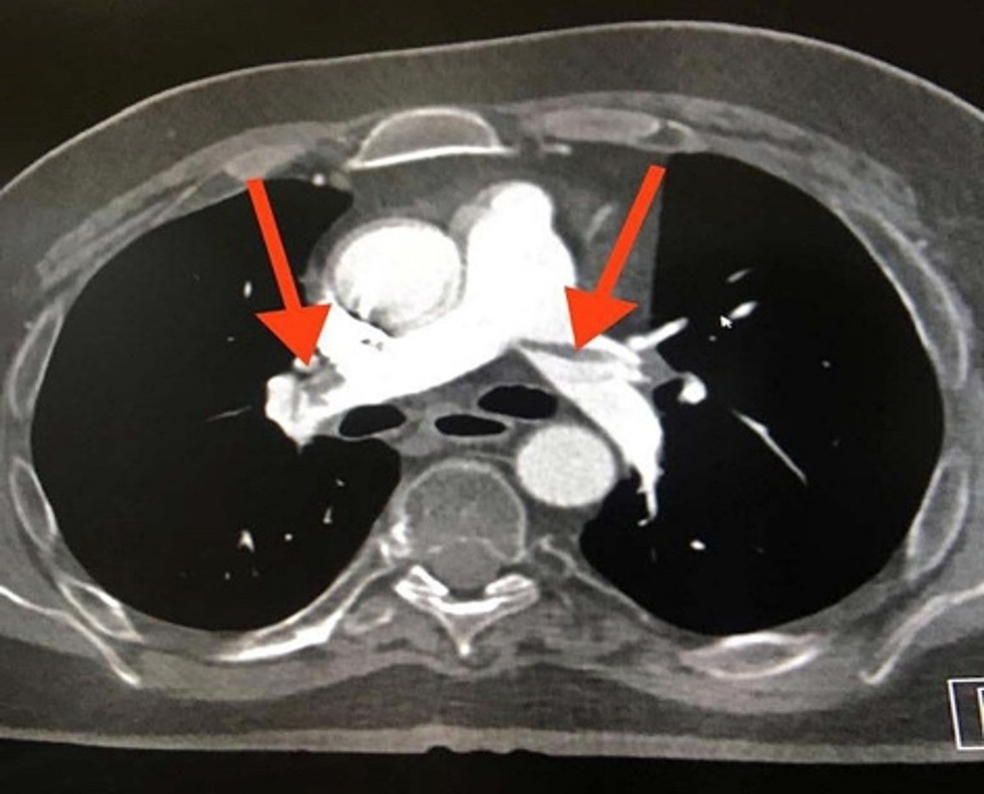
A cut of CT pulmonary angiography image showing filling defects in the pulmonary vasculature. A saddle embolus in the pulmonary artery extending into the left and right main pulmonary artery. Large embolus of interlobar, lobar, segmental, subsegmental left and right pulmonary arteries
